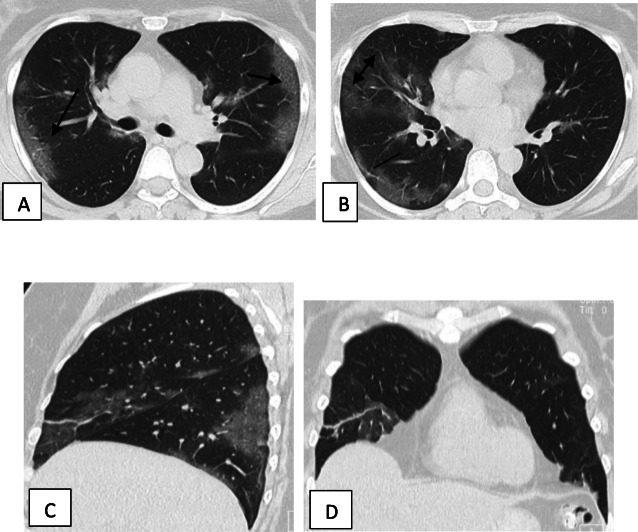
Unenhanced chest CT of a 43-year-old female patient with COVID-19. a, b Axial lung window images show bilateral subpleural areas of CP [single head arrows], subpleural and broncho-vascular areas of ground glassing opacity [double head arrow], and fibrosis [line]. c sagittal and d coronal reformatted. CT score is 11
